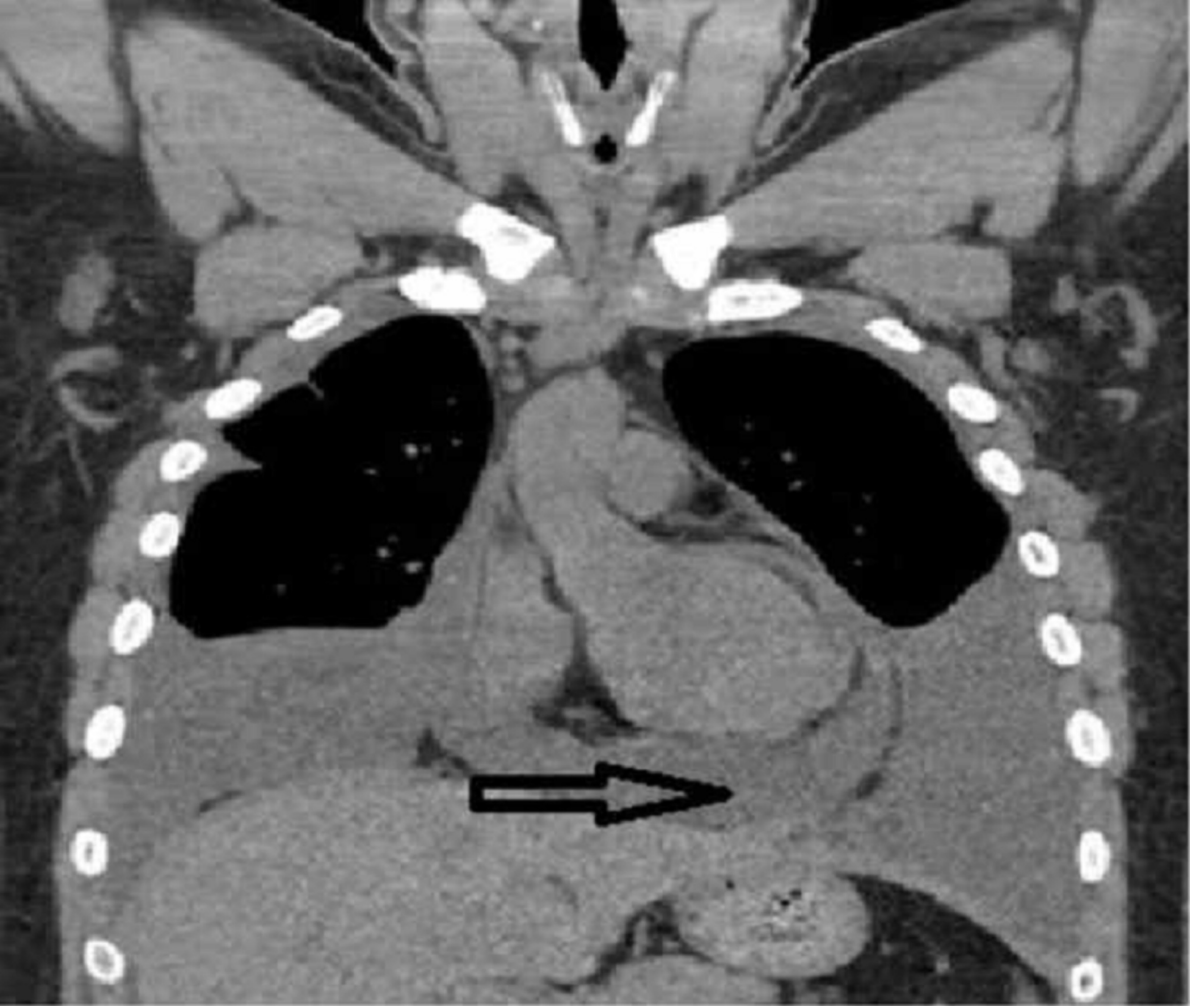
Computed tomography of chest (coronal view) showing pericardial effusion and bilateral pleural effusion Arrows pointing pleural and pericardial effusion
